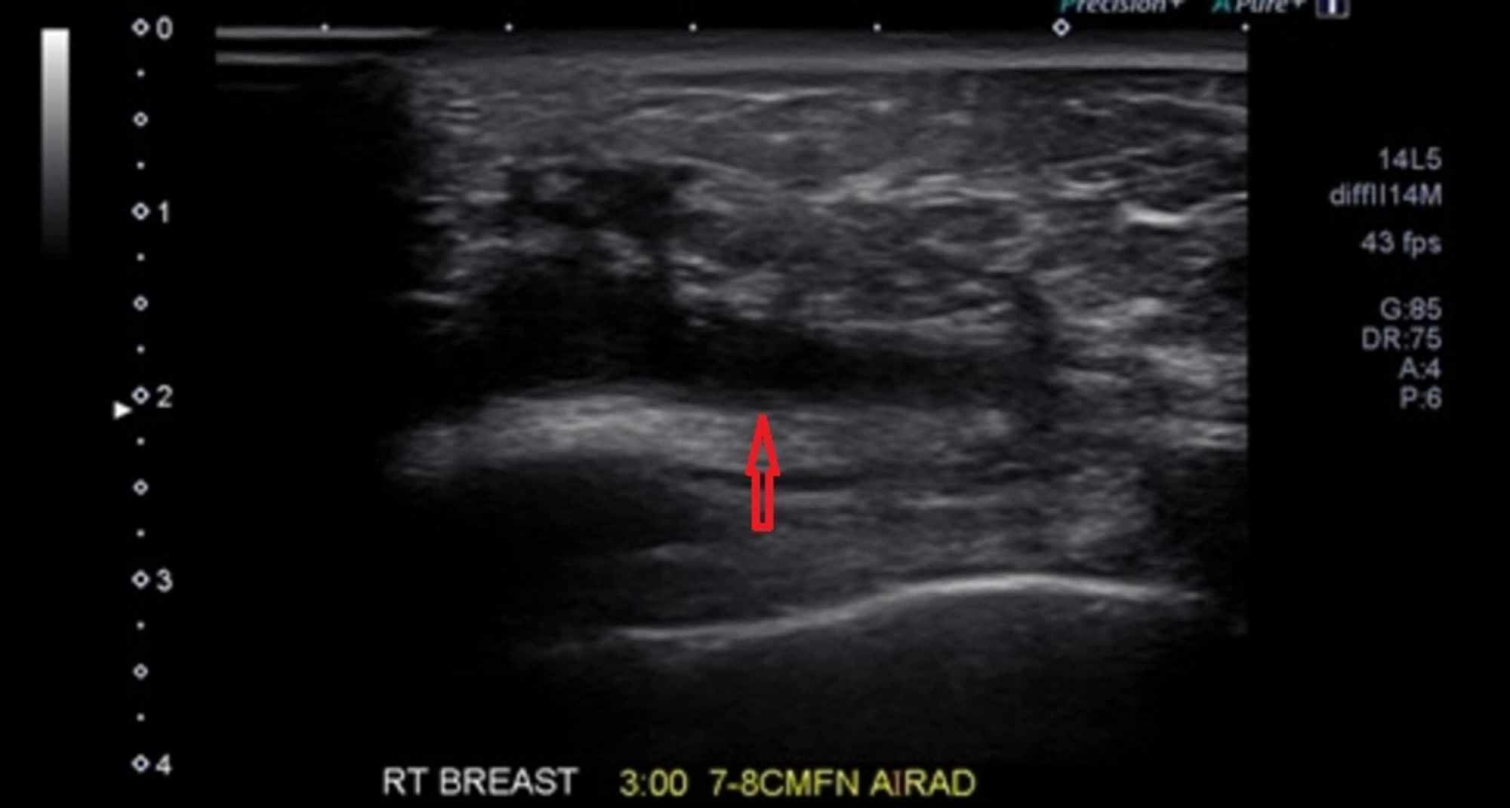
Breast ultrasound Breast ultrasound (US) post incision and drainage (I&D) shows resolution of parenchymal fluid and confirmation of a persistent path between the I&D site and the biopsied granulomatous mastitis lesion (red arrow).
